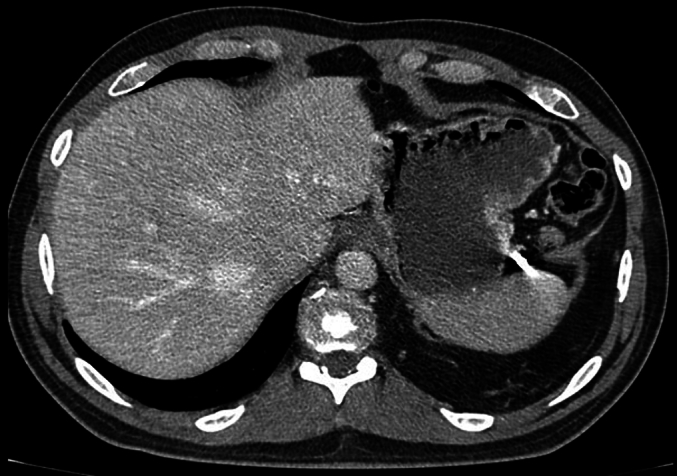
CT-thor/abd. with IV contrast, transverse cross-section.
